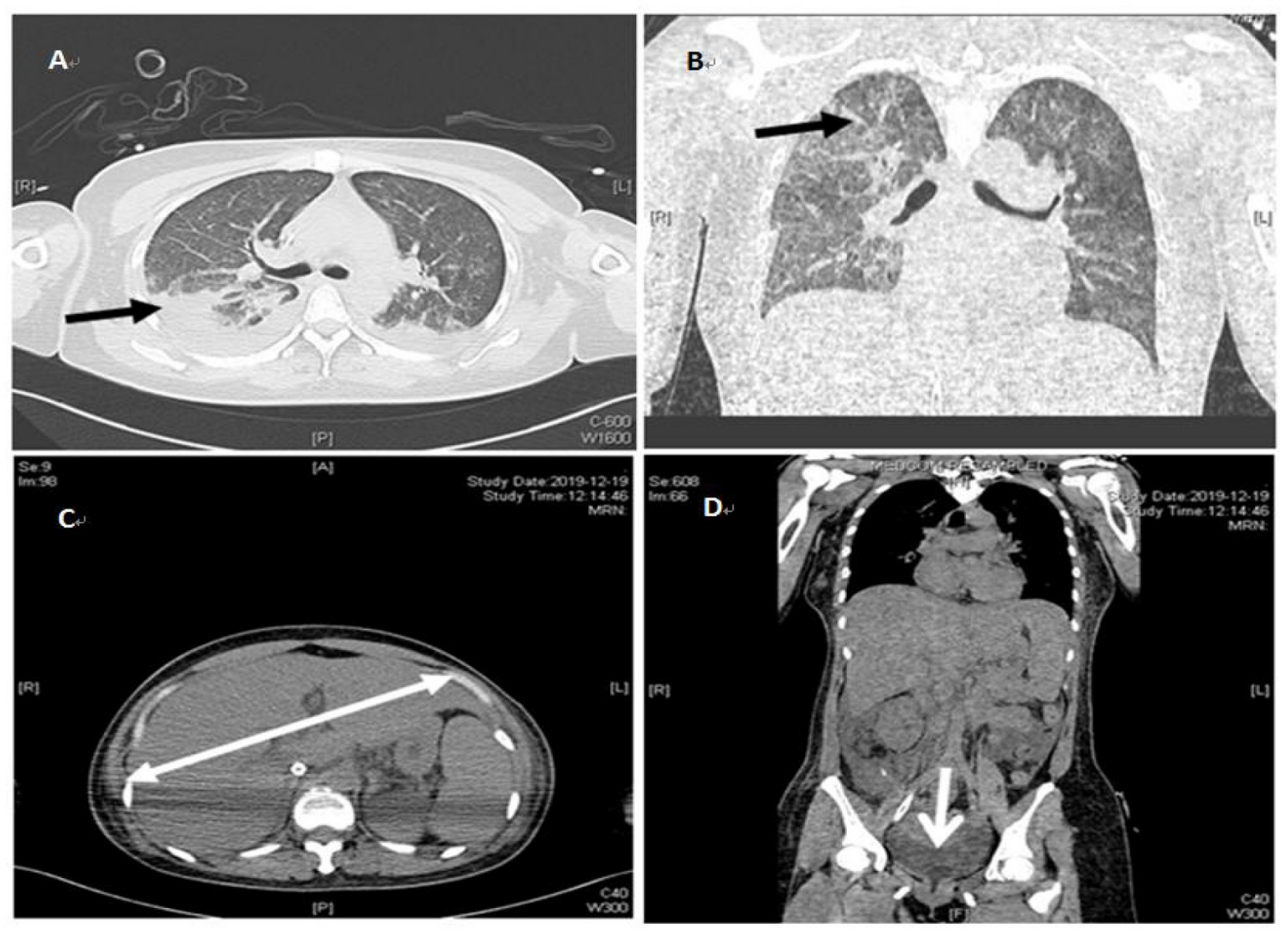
Repeat chest and abdominal CT on December 19, 2019. (A) indicates pleural effusion (black arrows). (B) indicates patchy blurring (black arrows). (C) indicates the enlarged liver (white k arrows). (D) indicates pelvic effusion (white k arrows).
